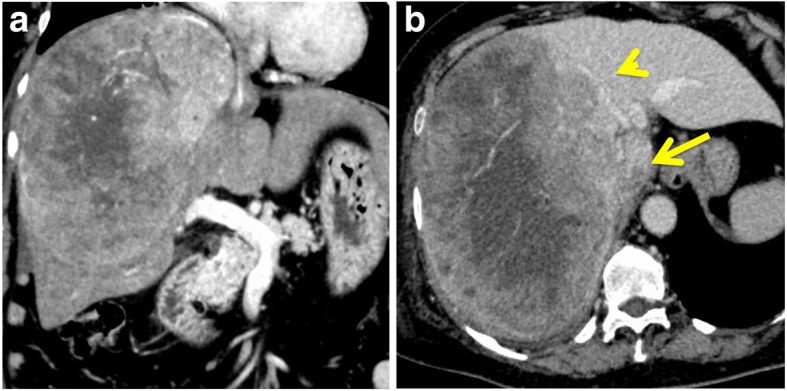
CT showed a huge tumor, 21 cm in diameter, in the right liver (a). The tumor compressed the middle hepatic vein (arrowhead) and inferior vena cava (arrow) (b)
